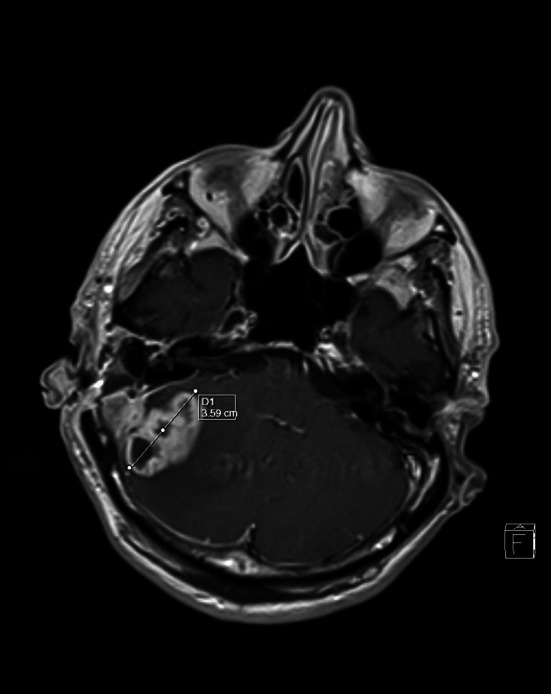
A 56-year-old patient with right-sided chronic otitis media with cholesteatoma complicated with a cerebellar abscessus. Left ear after radical mastoidectomy (MRI)
